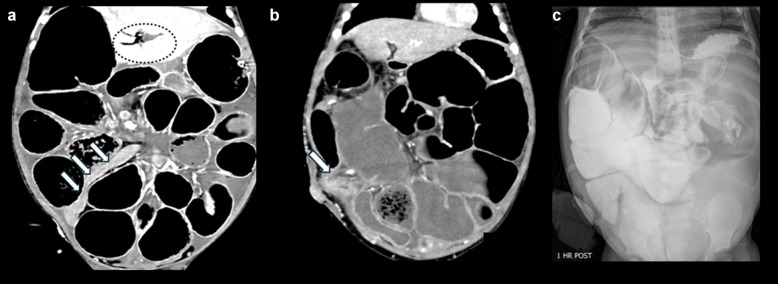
a Coronal CT abdomen/pelvis with IV contrast images in a 19-month-old girl with MMIHS who presented with abdominal distension and decreased ostomy output. There is portal venous gas (*circle*) and decompressed bowel loops directly upstream to the diverting ostomy (*white arrows*) with proximal bowel dilation, concerning for mechanical obstruction. The patient was found to have a dense adhesion twisting the ileostomy below the level of the fascia. There was bowel hypoperfusion without necrotic bowel. 5 cm of small bowel was resected, and the ostomy was revised. (b) Coronal CT abdomen/pelvis with IV contrast image obtained in the same patient 1 year later, after she presented with abdominal distension. The distal end of the ileostomy is decompressed (*arrow*). However, a small bowel follow-through (c) showed contrast extending to the ostomy bag, and therefore, the findings argued against obstruction. An ileoscopy performed soon after the exams demonstrated a normal appearance of the ileum, and stool contents were flushed
